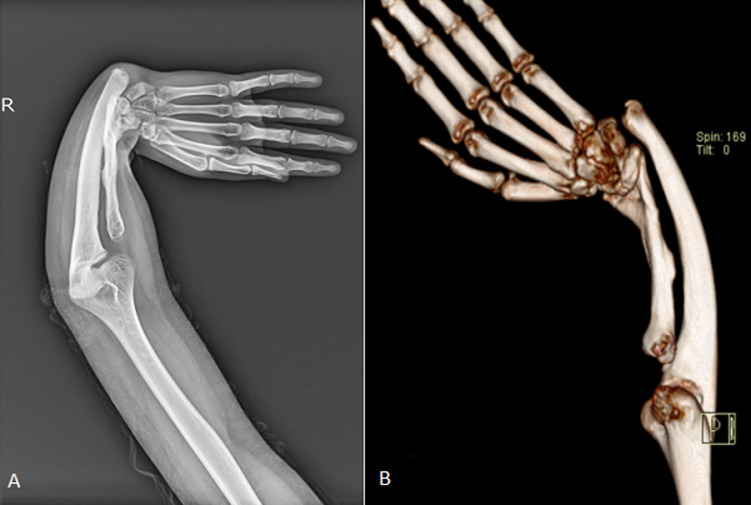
X-ray antero-posterior view (A) and computed tomography 3D reconstruction (B) of right forearm showing grossly shortened radius, deformed radius and ulna with radio ulnar dislocation
